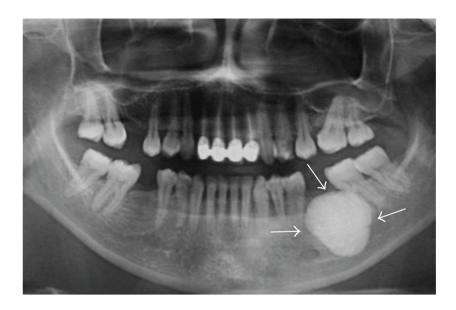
Panoramic radiograph showing a solitary, round, 3 × 3 cm well-defined radio-opaque mass without a radiolucent rim on the left side of the body of the mandible. The lesion extended distally of the second premolar till the mesial aspect of the second molar distal root.
